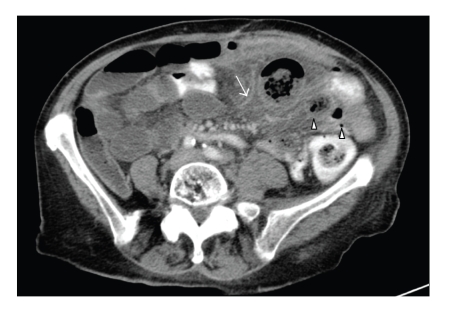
Transverse CT section through the lower abdomen reveals localised inflammatory changes in the left mesenteric root (arrow). Centrally within this pseudomass, several air containing small-bowel diverticula can be noted (arrow heads).
